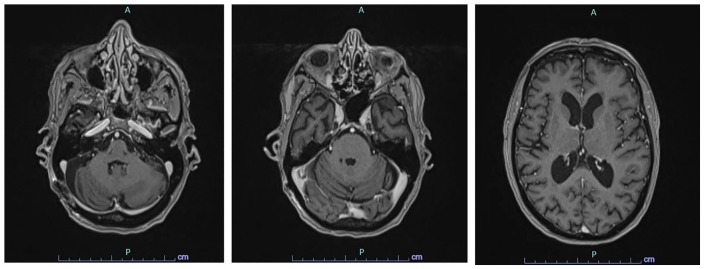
Brain MRI after 6 cycles of chemotherapy completion showing interval
